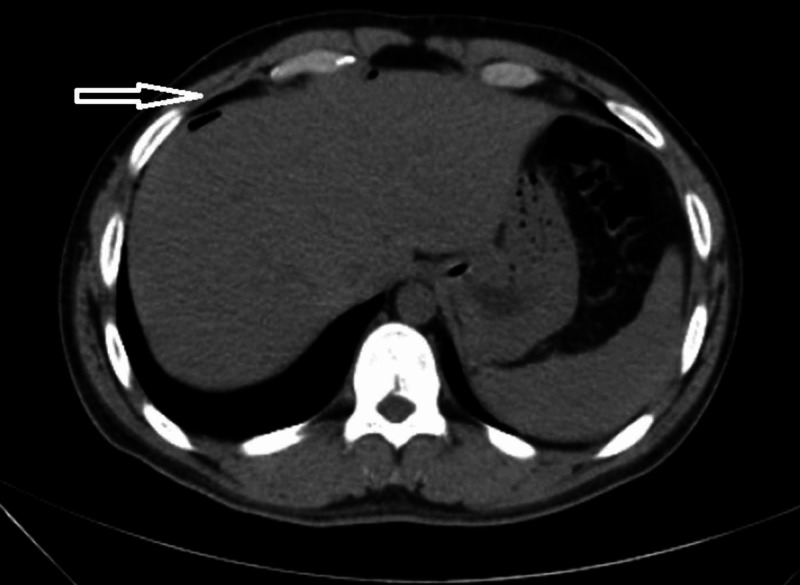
CT abdomen showing air pocket in subdiaphragmatic region suggestive of pneumoperitoneum (depicted by arrow).
